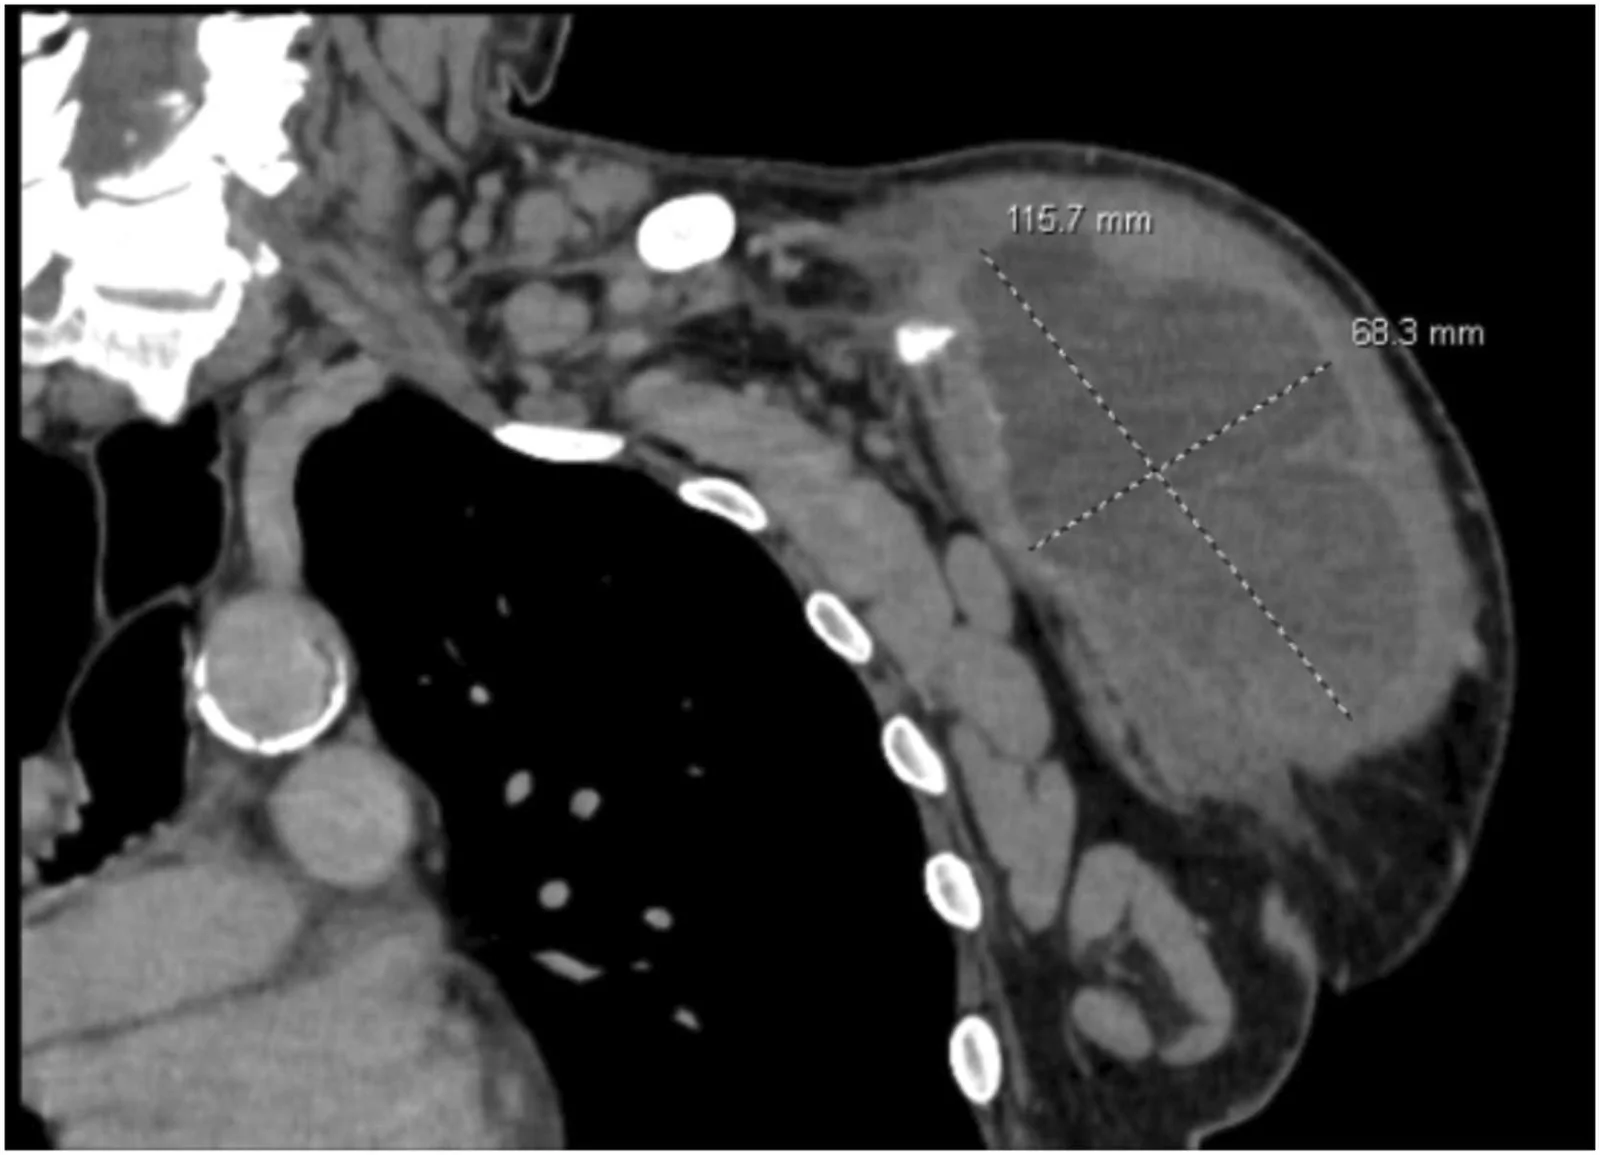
Contrast-enhanced CT of the left shoulder demonstrating a moderate glenohumeral joint effusion with severe joint space narrowing and cortical erosion of the humeral head and inferior glenoid, consistent with septic arthritis. A complex multiloculated, peripherally enhancing fluid collection extends into the deltoid, triceps, and supraspinatus muscles, compatible with intramuscular abscesses. Diffuse edema of the rotator cuff musculature is also present.
